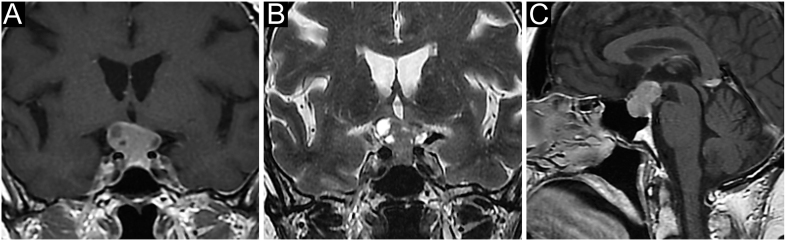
An invasive ACTH-secreting macrotumour in a 76-year-old woman. This patient was referred for the endocrinological evaluation of a sellar/suprasellar mass, incidentally revealed by cerebral imaging after a domestic fall complicated by epistaxis. The patient complained of unexplored weight gain (7–8 kg over the past 18 months) and increased pharmacological requirement for the treatment of hypertension. Mild Cushingoid features were observed, with facial rounding, central obesity, a BMI of 34.2 kg/m^2^ and peripheral muscle wasting. ACTH-dependent hypercortisolism was confirmed by elevated urinary free cortisol levels (1.7 × ULN), lack of suppression of serum cortisol following overnight 1 mg dexamethasone (7.5 μg/dL) and elevated ACTH concentration (142.7 pg/mL). A pituitary macrotumour with suprasellar extension and cystic changes was found at pituitary MRI (A, B, C). Transsphenoidal surgery was performed and pathological examination confirmed diffuse ACTH immunoreactivity with low Ki-67 (1%) in the absence of p53 expression. Post-operative headache occurred due to a modest suprasellar haematoma, which progressively regressed. Bio-clinical post-operative remission of hypercortisolism occurred despite a small tumour remnant. Three years later, a mild bio-clinical recurrence of hypercortisolism occurred with tumour regrowth. The patient received stereotactic radiotherapy and cabergoline (CAB, up to 2 mg/week) with tumour stabilization and bio-clinical normalization of hypercortisolism. Three years later, escape to CAB led to introduction of low-dose osilodrostat (up to 1 mg × 2 daily) with a good tolerance and control of hypercortisolism. However, very last MRI follow-up is suggestive for disease progression, which is under multidisciplinary re-evaluation. (A) Coronal contrast-enhanced T1-weighted scan, (B) coronal T2-weighted scan and (C) sagittal contrast-enhanced T1-weighted scan.
